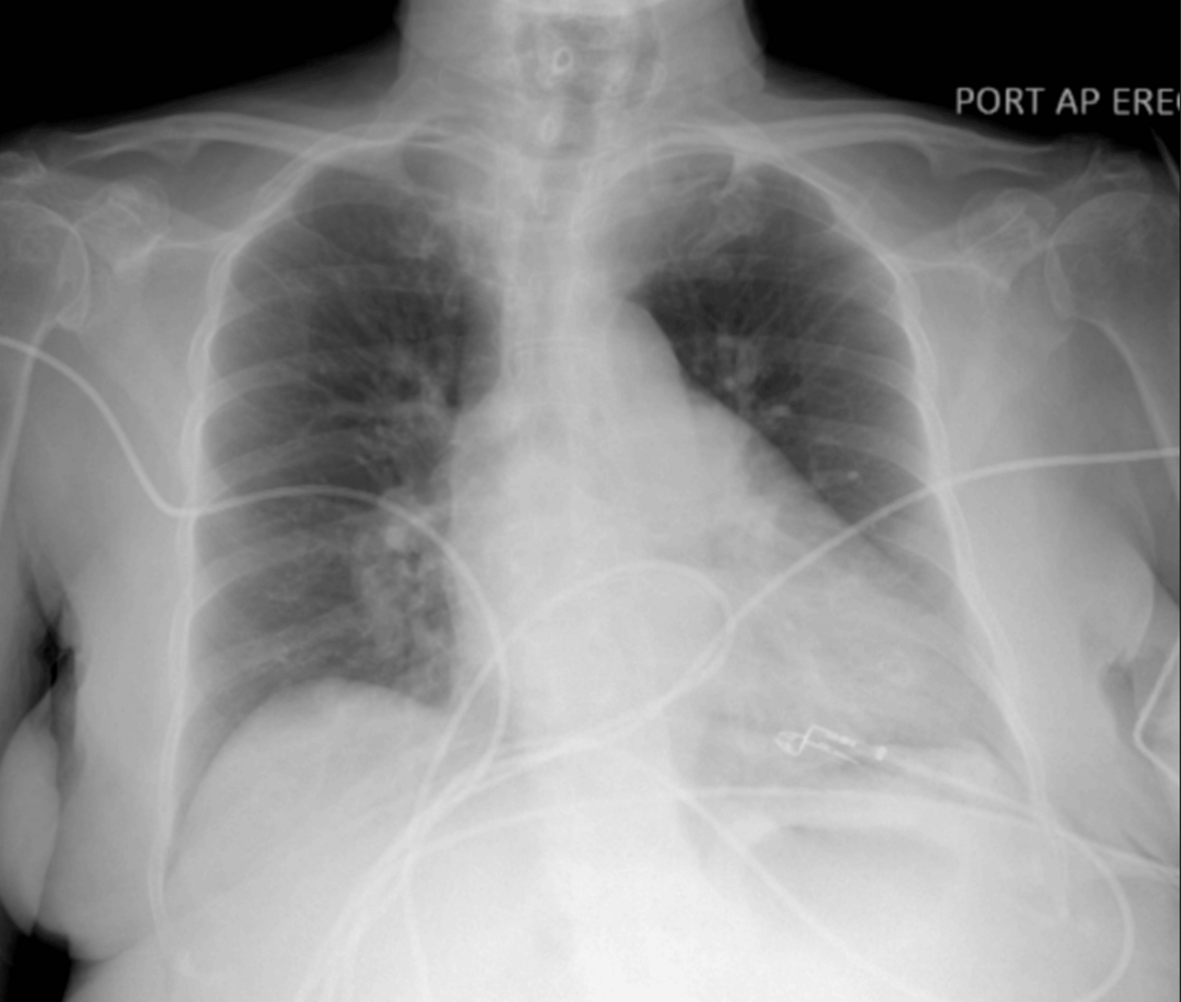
CXR showing mild bilateral vascular congestion. No gas under the diaphragm was noted CXR: Chest X-ray
